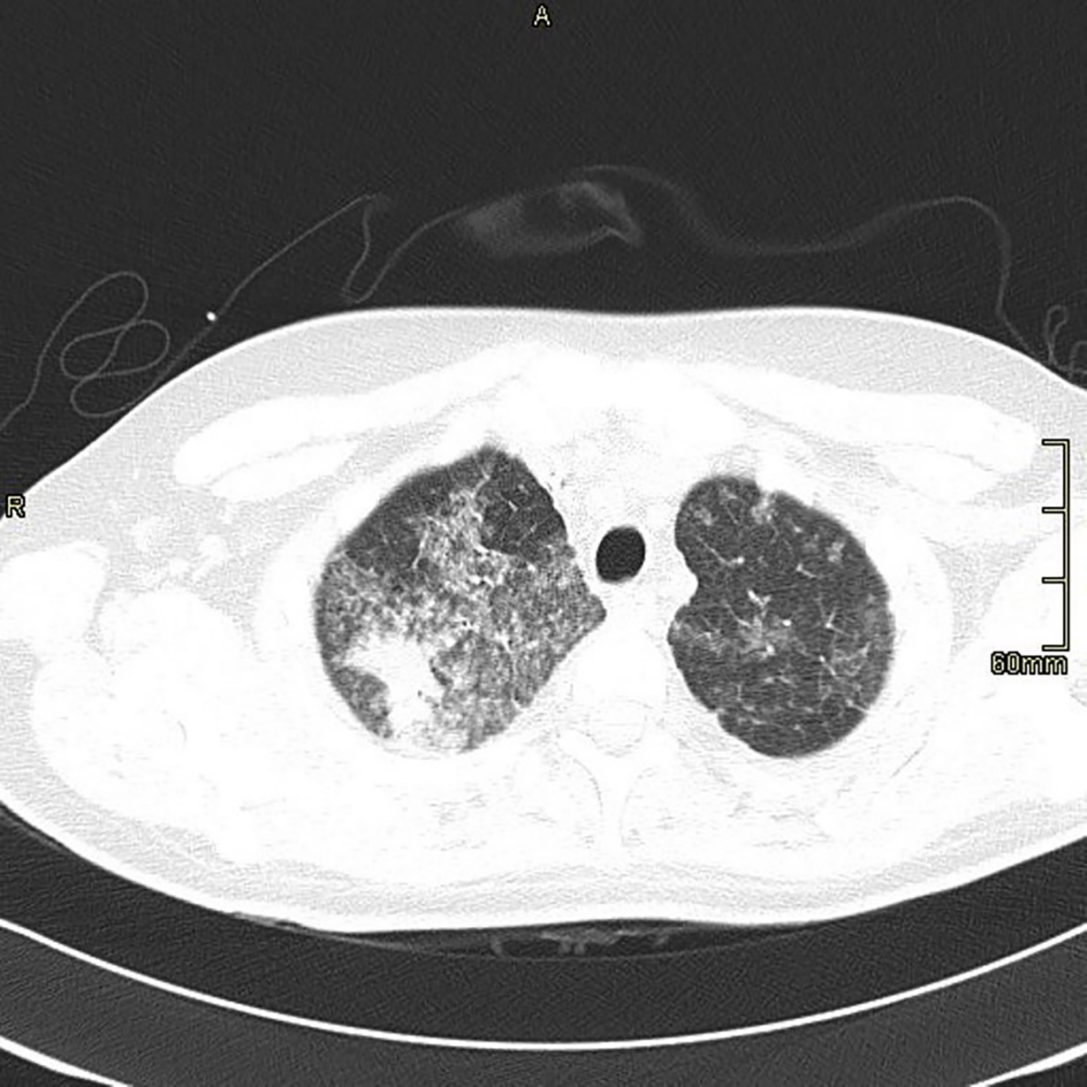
CT chest showing patchy ground glass opacities with right apical consolidation.
